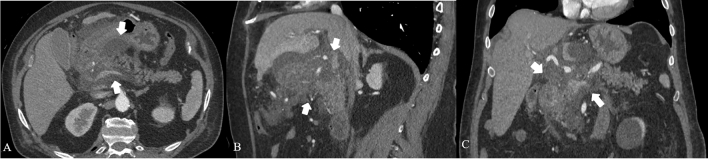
ANCs in biliary AP in a 74 years-old man. The contrast-enhanced CT axial scan (a) shows the presence of an acute necrotic fluid collection (ANCs) interesting the head and the body of the pancreatic gland (white arrows), extending in the fat surrounding the gland up to the gastrohepatic ligament. The sagittal MPR (b) and coronal (c) better show the extention of the collection
